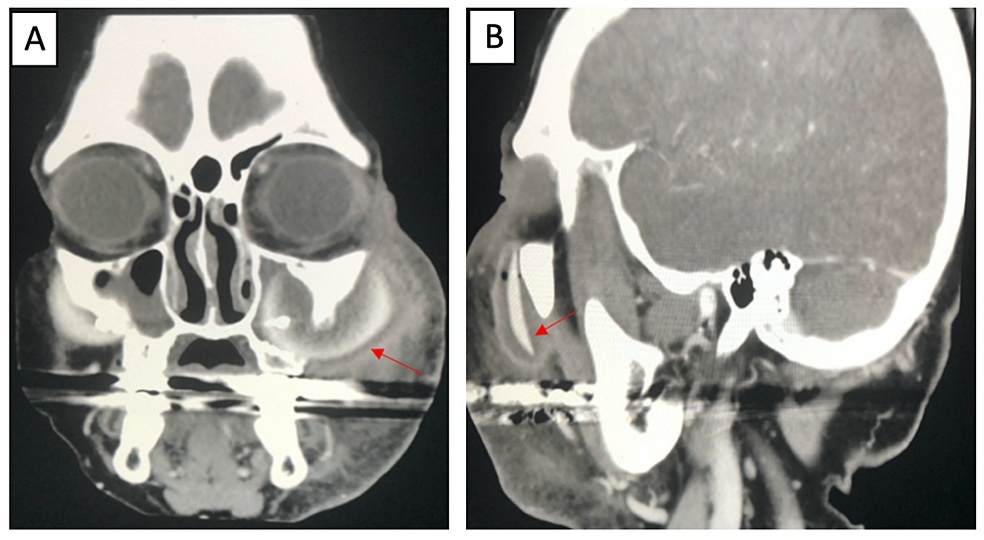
Enhanced CT in coronal (A) and sagittal (B) views. Enhanced CT displaying abscess formation in coronal view (A) around a left malar implant penetrating into the left maxillary sinus in sagittal view (B).
